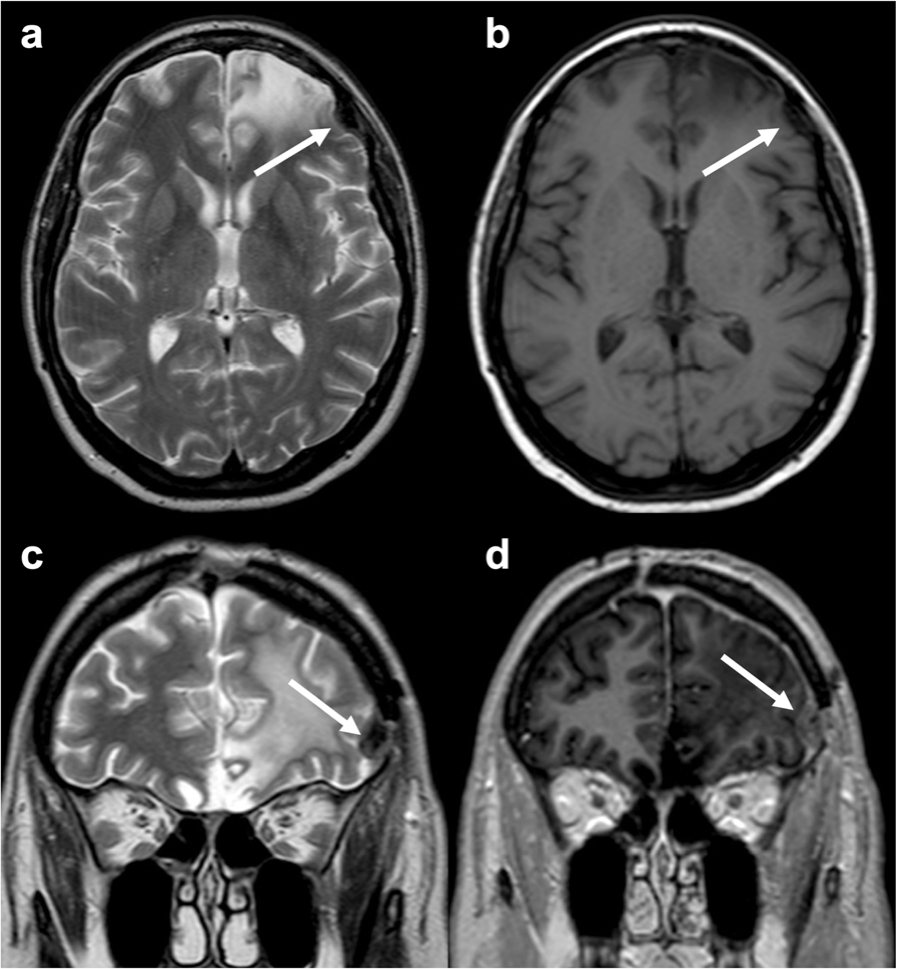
Bone wax. Axial T2-weighted (a) and T1-weighted (b) and coronal T2-weighted (c) and post-contrast T1-weighted (d) images depicting burr hole bone wax, hypointense on both T1- and T2-weighted sequences (arrow in a, b, and c). Reactive enhancement is evident on post-contrast T1-weighted image (arrow in d)
